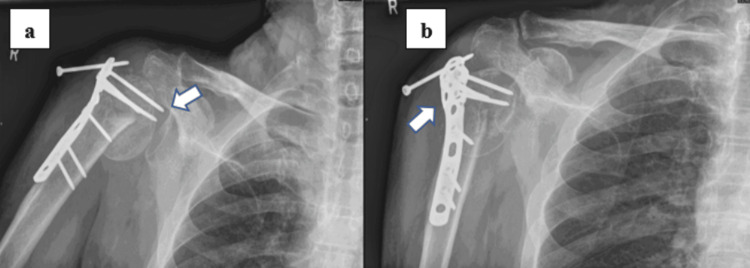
Radiographs of the right shoulder taken during the first clinic visit, four months after the surgery. (a) Anteroposterior radiograph of right shoulder demonstrating screw penetration (arrow) and collapse of the humeral head. (b) Lateral radiograph of the right shoulder shows a locking screw backout (arrow) and prominent fracture line.
